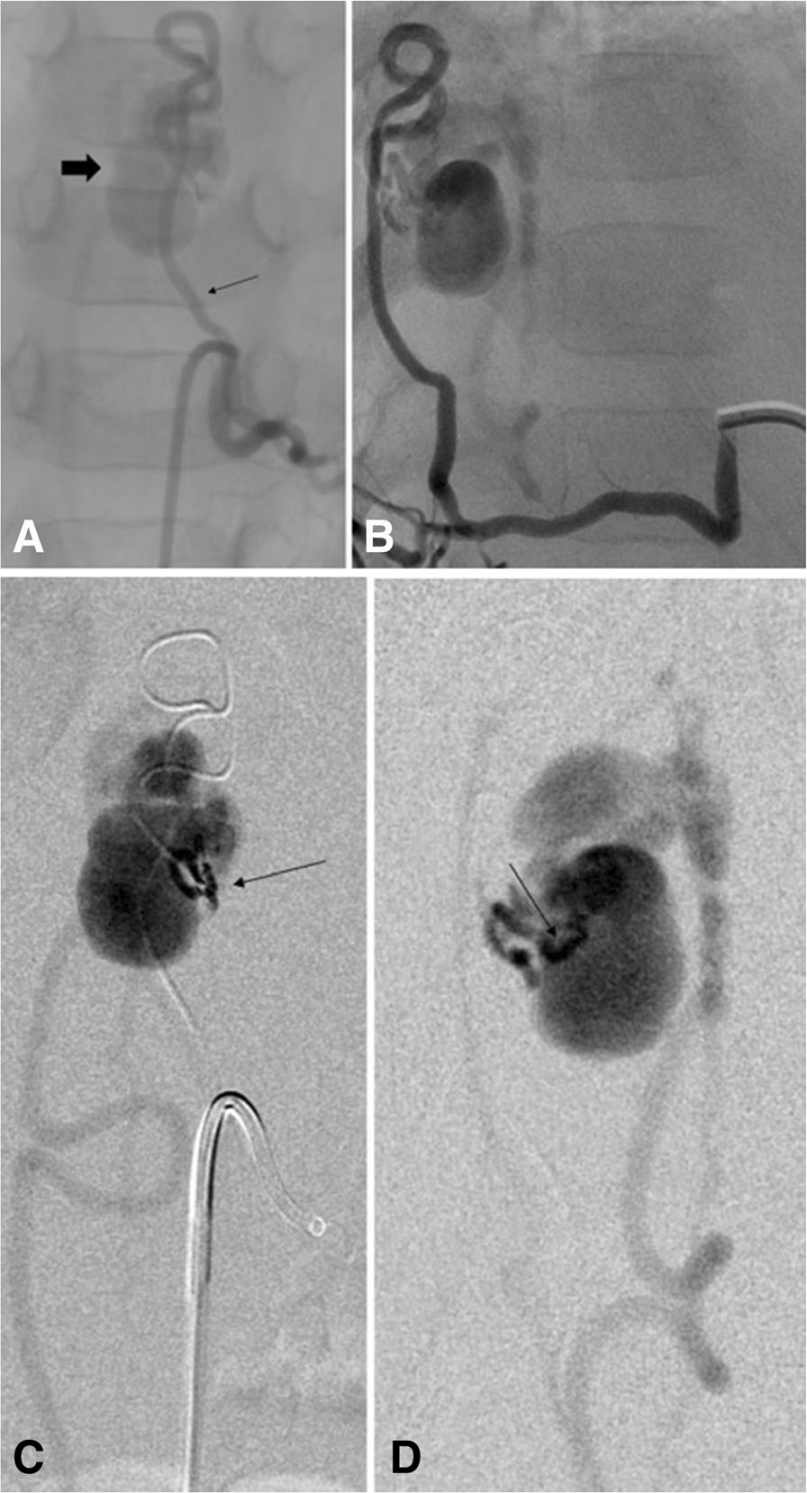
A and B 3 yo male with left perimedullary fistula. Left T10 intercostal angiogram in the AP and lateral projections shows an enlarged radiculomedullary artery (small arrow) filling a venous varix (thick black arrow) corresponding to the CTA and MRI images. C and D Selective microcatheter injection in the AP and lateral projections (black arrow) in the venous varix shows filling of the varix and surrounding perimedullary venous plexus
